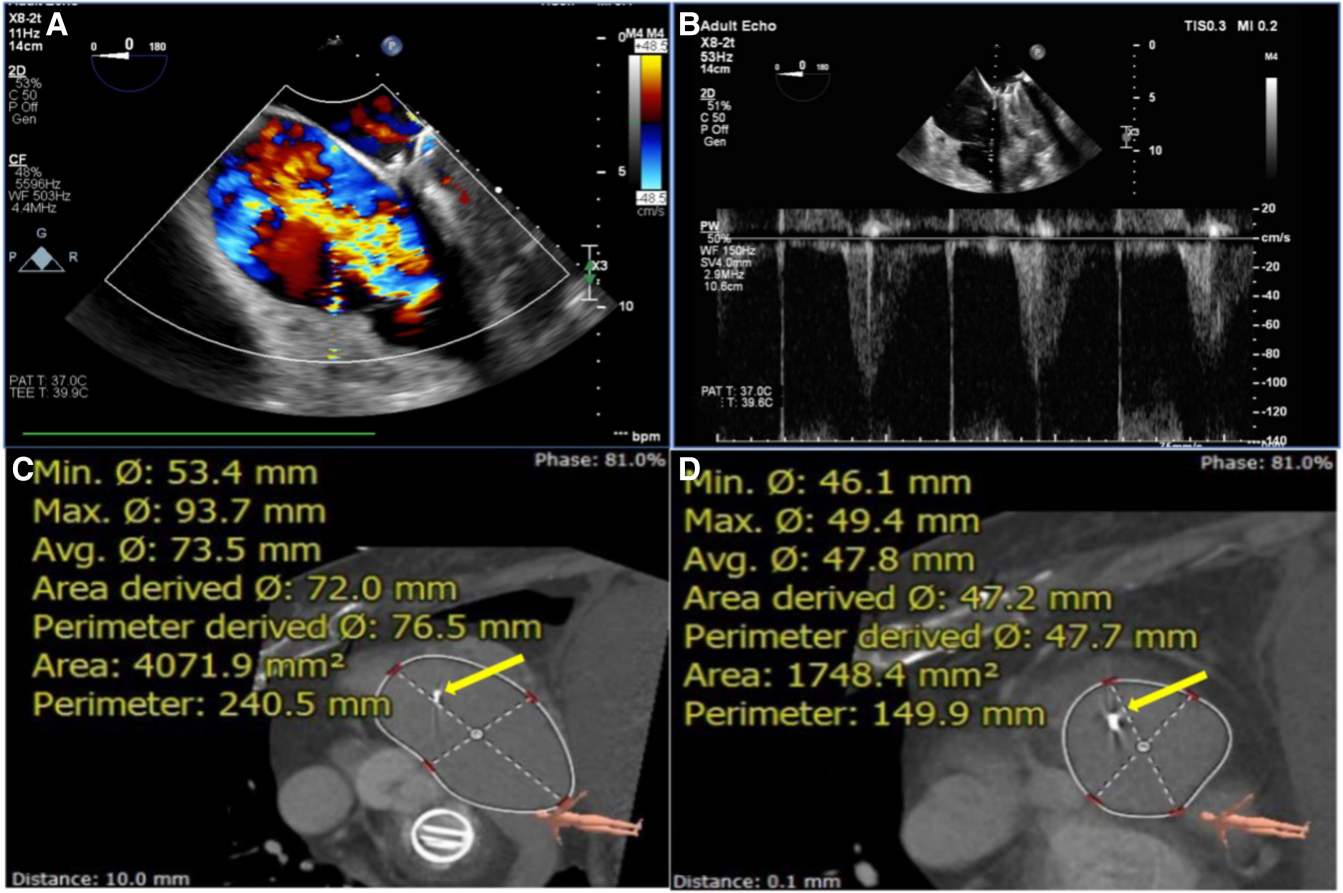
Assessment of TEE and CTA before TTVR evaluated the status of TV (e.g., data for patient #6) assessment of RA and RV using circle cardiovascular imaging CVI42 software. (A) TEE showed large regurgitation on TV. (B) The maximum velocity of TV was up to 1.1 m/s. (C, D) The area of the RA was 4071.9 mm^2^, and the area of the RV was 1748.4 mm^2^. The yellow arrows indicate the lead of the permanent pacemaker. TEE, transesophageal echocardiography; CTA, computed tomography angiography; TTVR, transcatheter tricuspid valve replacement; TV, tricuspid valve; RA, right atrium; RV, right ventricle; TA, tricuspid annulus.
